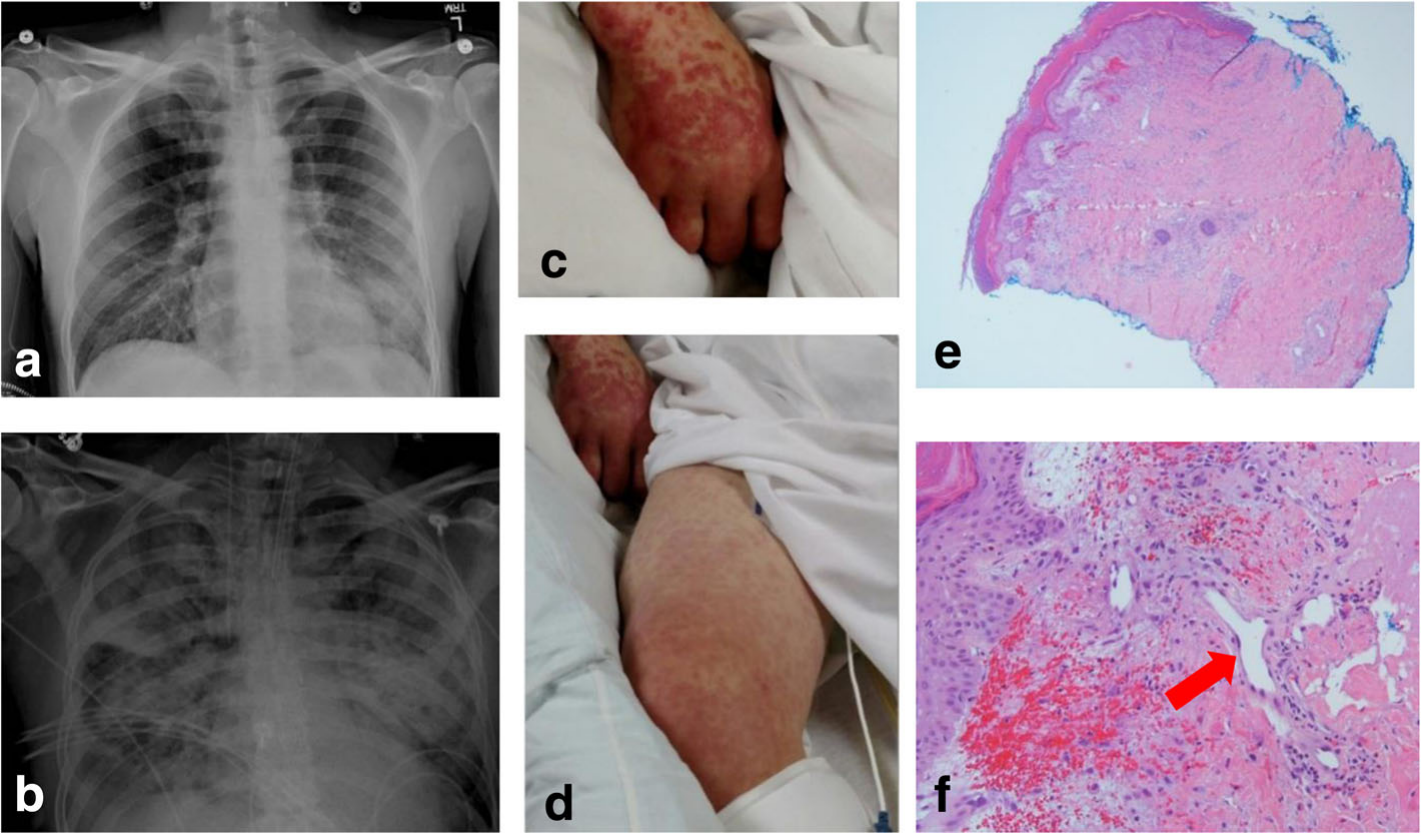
Clinical and imaging manifestation of the case. a Chest X-Ray on day 1 showing mild left lower lobe airspace opacity; b Chest X-Ray on day 3 showing diffuse pulmonary infiltrate; c&d Diffuse rash on day 8; e 3 mm punch biopsy of the dorsum of the right hand; f Absence of fibrin and microthrombi deposition in the vessel lumen (arrow)
